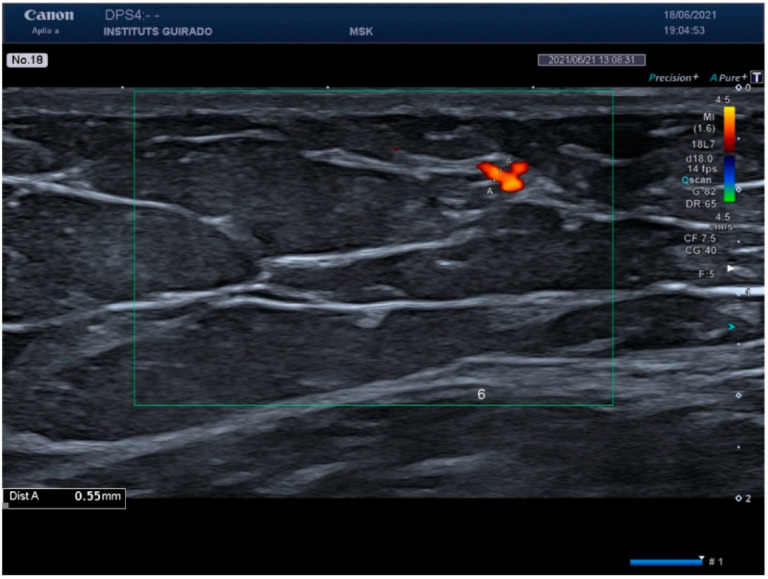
Vessel diameter measurement—this figure presents the measurement of vessel diameters in healthy skin, highlighting the detection capabilities of the Doppler ultrasound.
